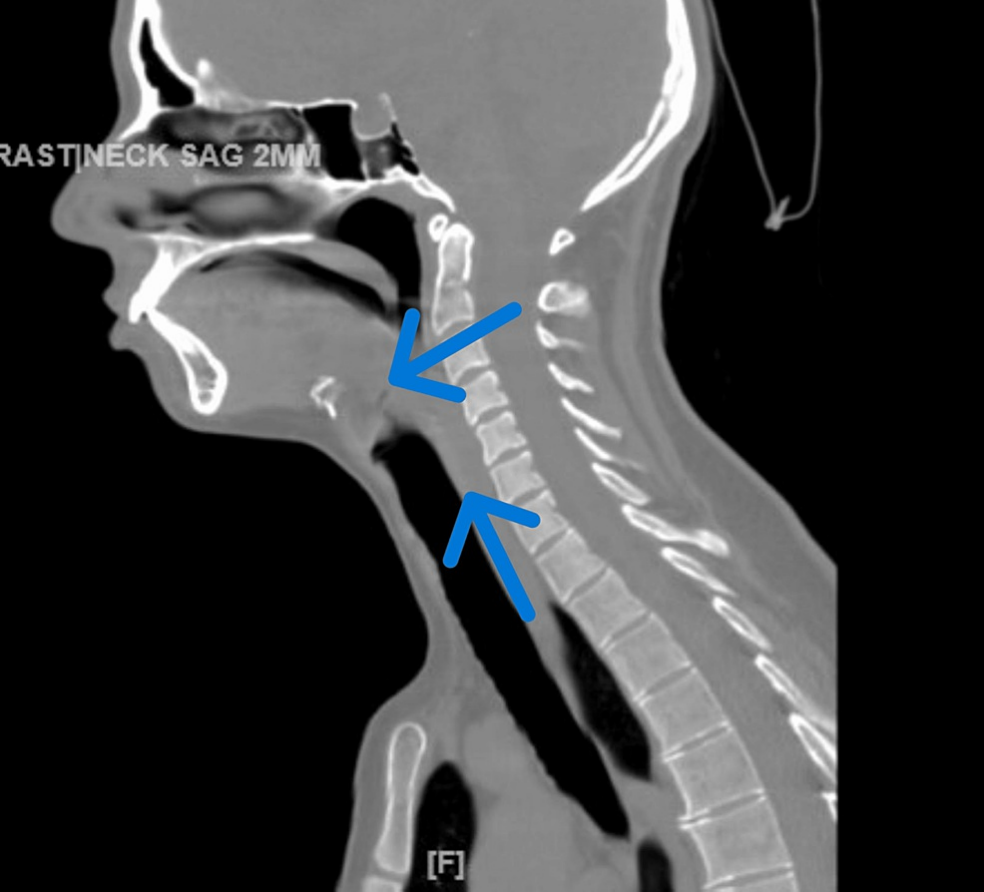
A CT scan of the soft tissues of the neck (without contrast) during transfer to the medical floor showed extensive soft tissue swelling within the larynx and hypopharynx
